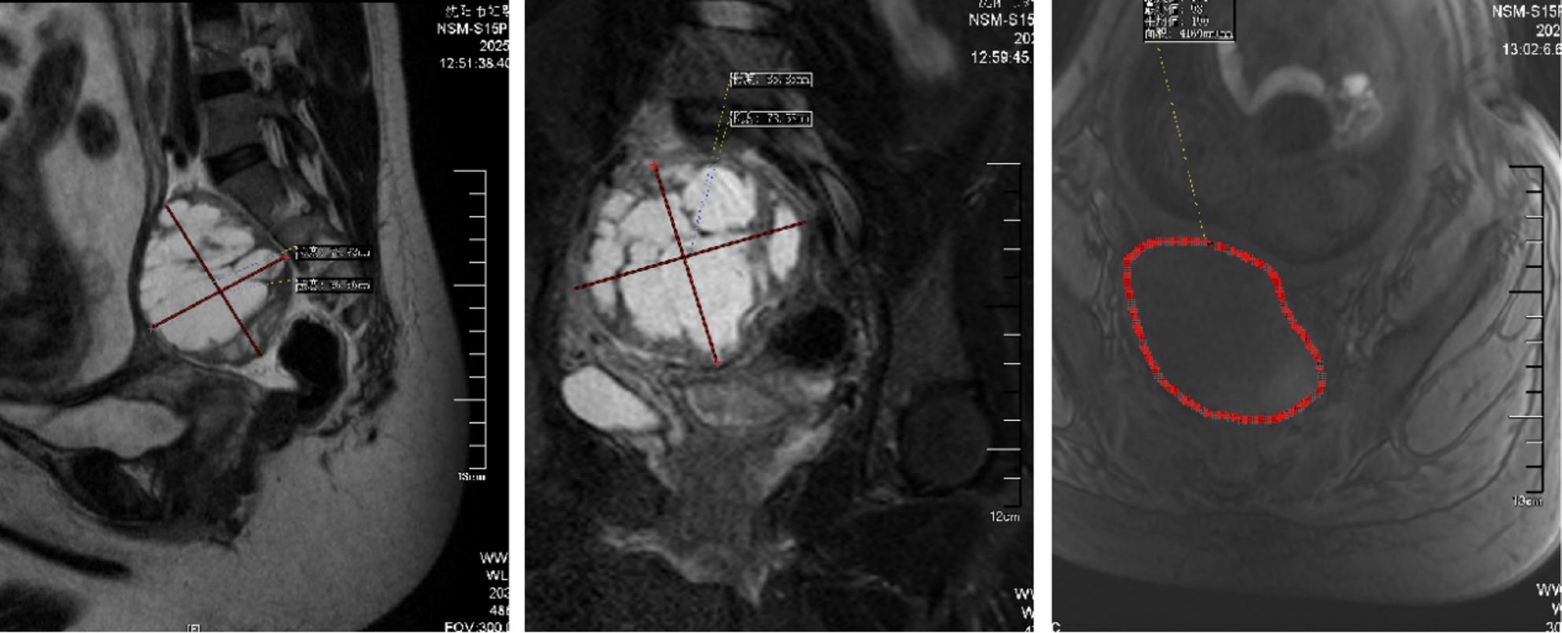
MRI on admission at 33 + 5 weeks of gestation. A cystic mass (85 mm × 73 mm × 66 mm) with mixed T1/T2 signals and septations is observed behind the cervix, suggestive of hemorrhage
